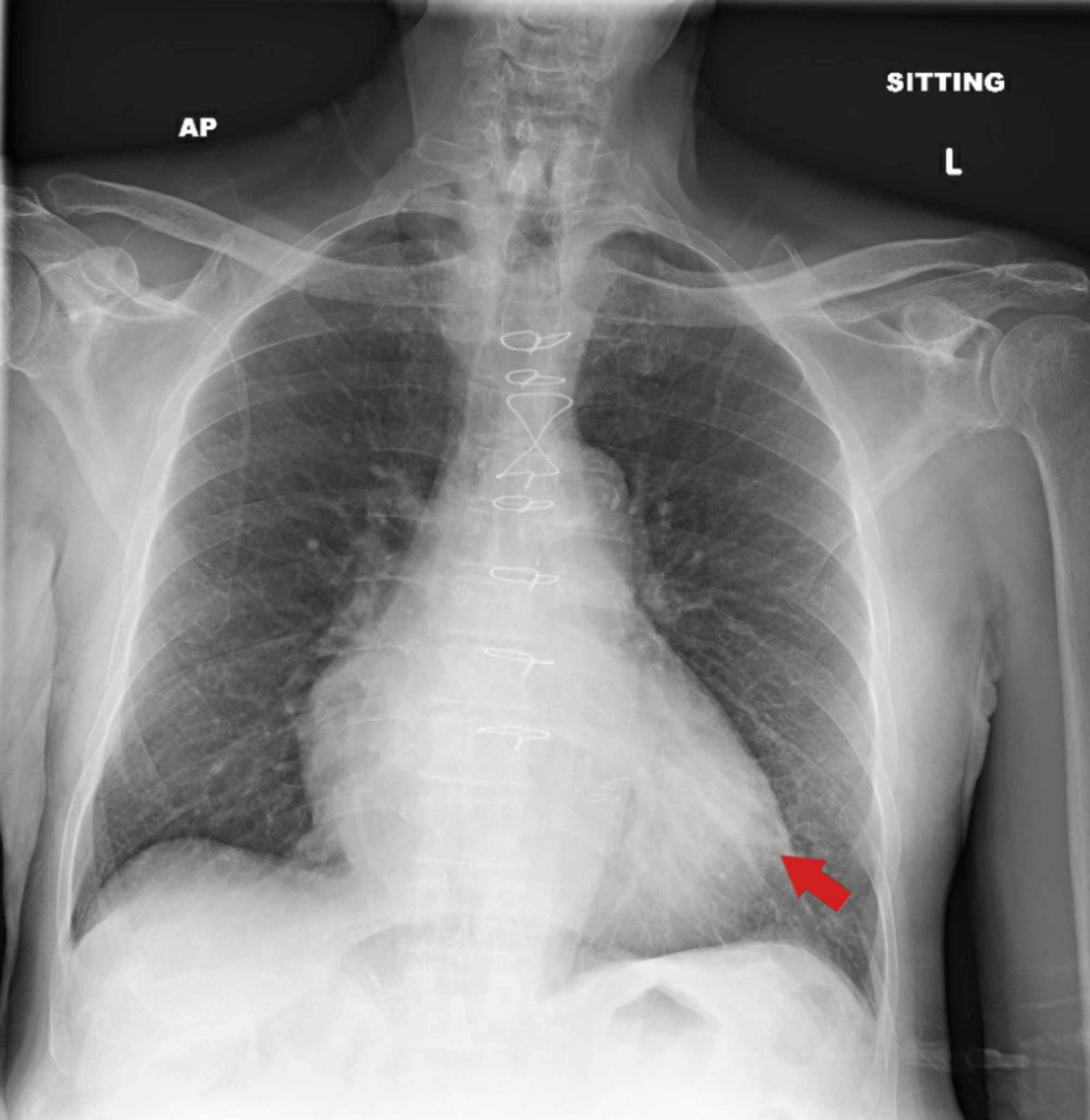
CXR on admission showed moderate cardiomegaly and no lobar consolidation. CXR, chest X-ray
